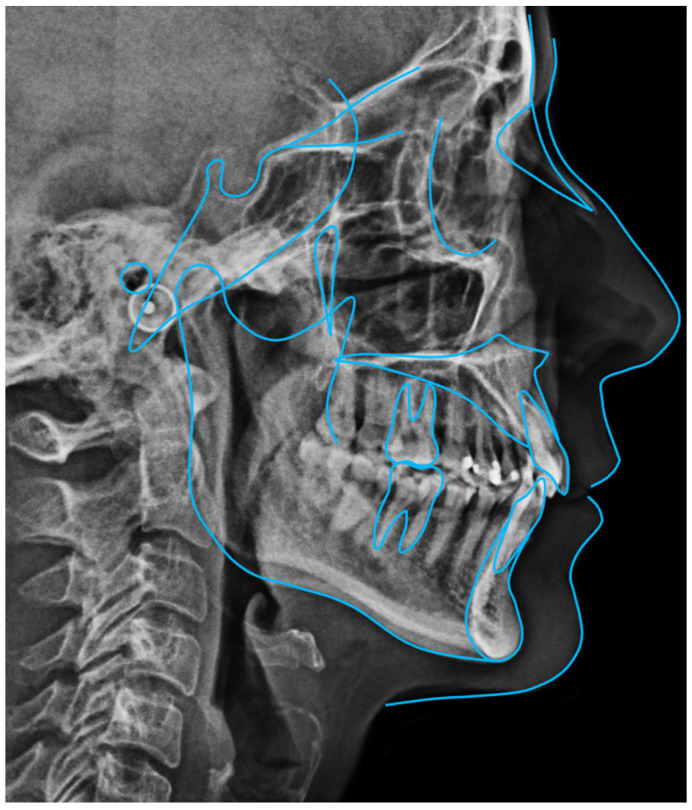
Initial cephalometric tracing of Clinical Case 1. The blue lines represent the cephalometric tracing overlaid on the radiographic image.
